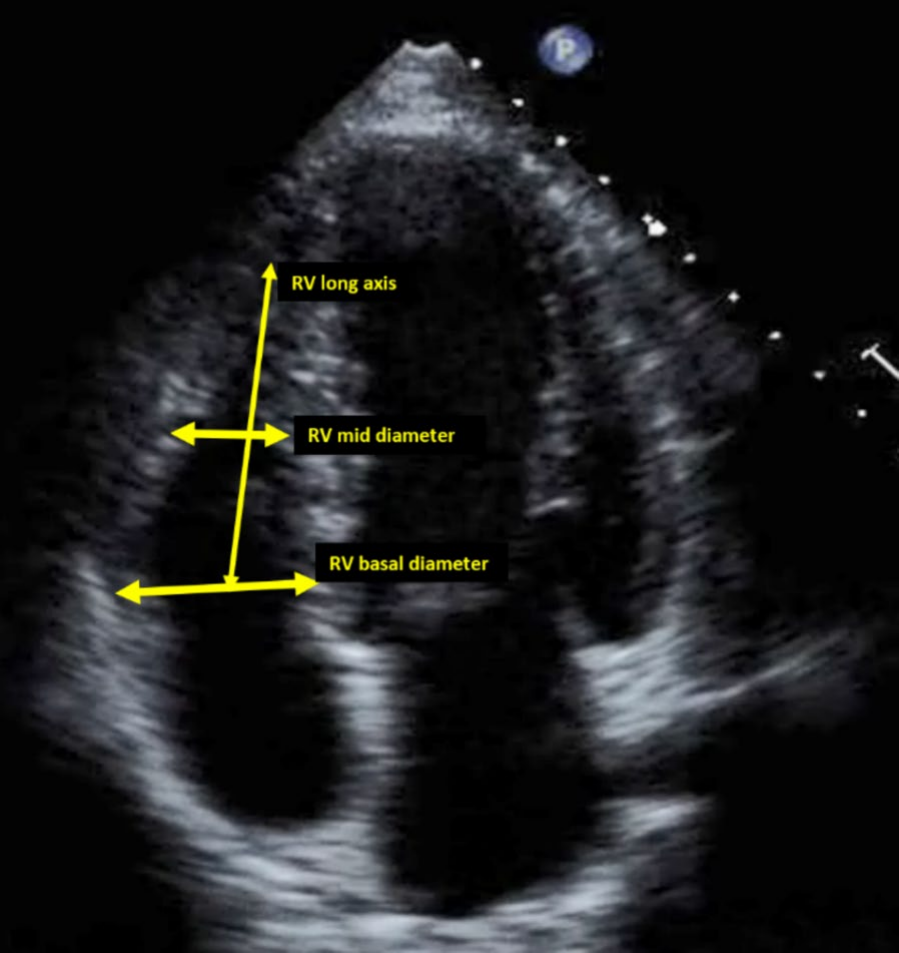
RV-focused apical four-chamber image showing measurement of RV long-axis and basal and mid-minor axis diameters
